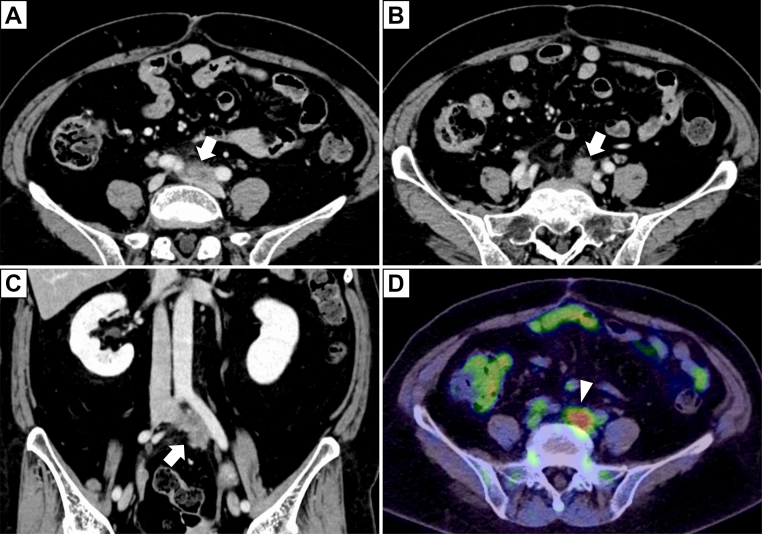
Preoperative image. Preoperative contrast-enhanced computed tomography (CT) and positron emission tomography-CT of the abdomen. (A and B) On the axial CT slice, enlargement of the left common iliac lymph node was noted (*white arrow*), with infiltration extending from the terminal aorta to the bifurcation of the left common iliac artery (CIA). (C) On the coronal CT slice, infiltration of the left common iliac lymph node into the left common iliac vein (CIV) was observed (*white arrow*). (D) Positron emission tomography-CT revealed accumulations in the enlarged common iliac lymph node (*white arrowhead*).
